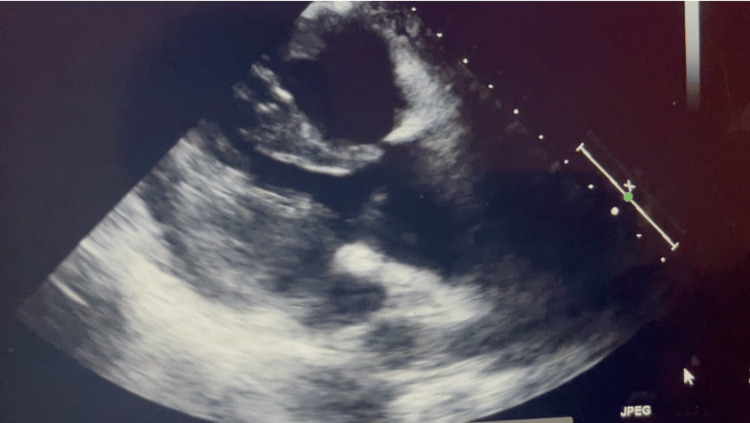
Two-dimensional echocardiography with an estimated left ventricular ejection fraction of 45-50% and grade 1 diastolic dysfunction, with aortic root dilation at 4 cm
